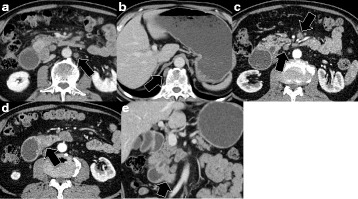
Enlarged para-aortic (a, *arrow*) and paravertebral lymph node (b, *arrow*), regional lymph nodes (c, *arrows*), and primary duodenal tumor (d, e, *arrows*) are detected by enhanced computed tomography
